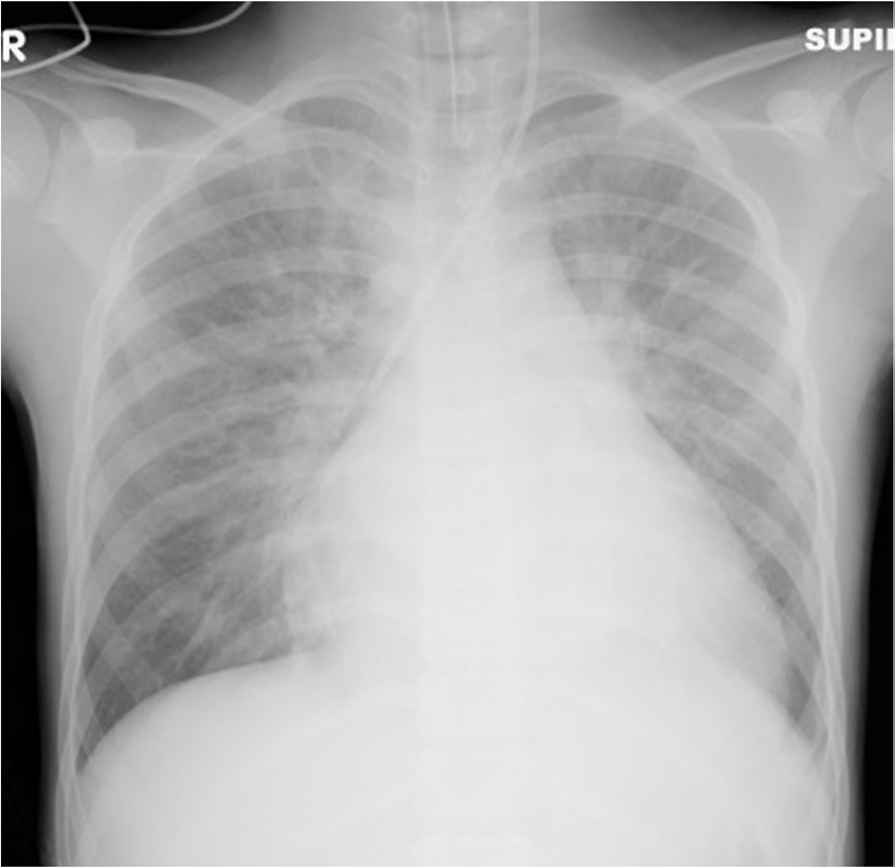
Chest radiograph on admission to ICU
